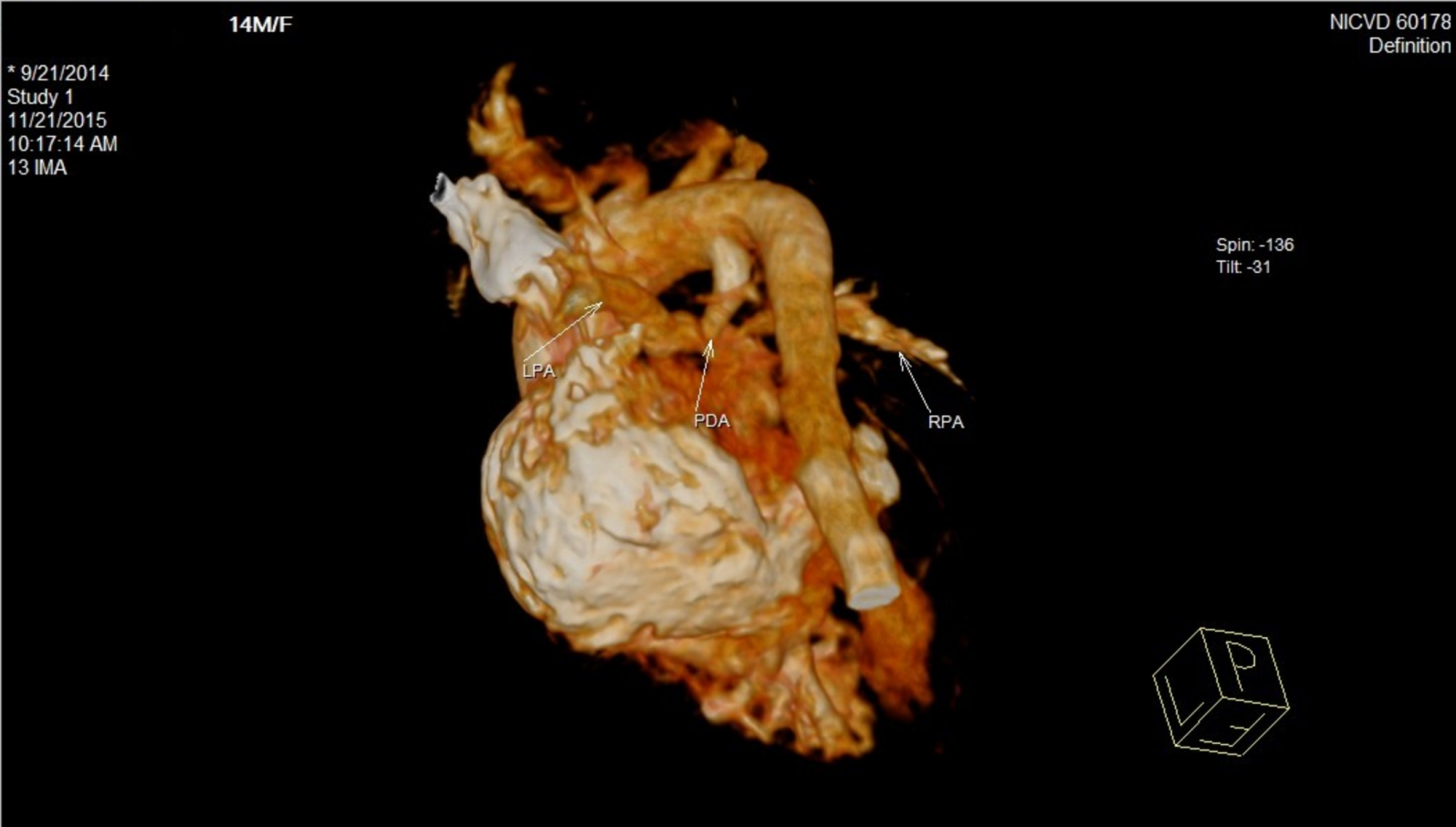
Cardiac CT scan showing patent ductus arteriosus in the patient RPA, right pulmonary artery; LPA, left pulmonary artery; PDA, patent ductus arteriosus
